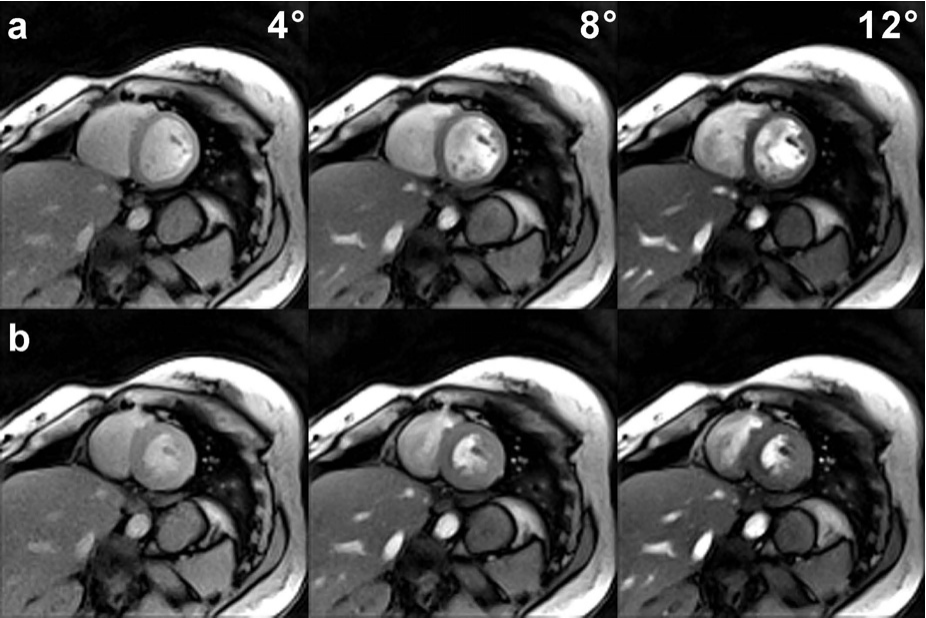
T1 contrast in real-time radial FLASH CMR. T1-weighted images (spoiled FLASH, TR/TE = 2.0/1.3 ms, 15 spokes, acquisition time 30 ms) with increasing T1 contrast as a function of flip angle (4°, 8°, 12°) for a short-axis view (2.0 mm resolution, 8 mm section thickness) during (a) diastole and (b) systole. The images were selected from respective real-time CMR movies reconstructed by nonlinear inversion.
